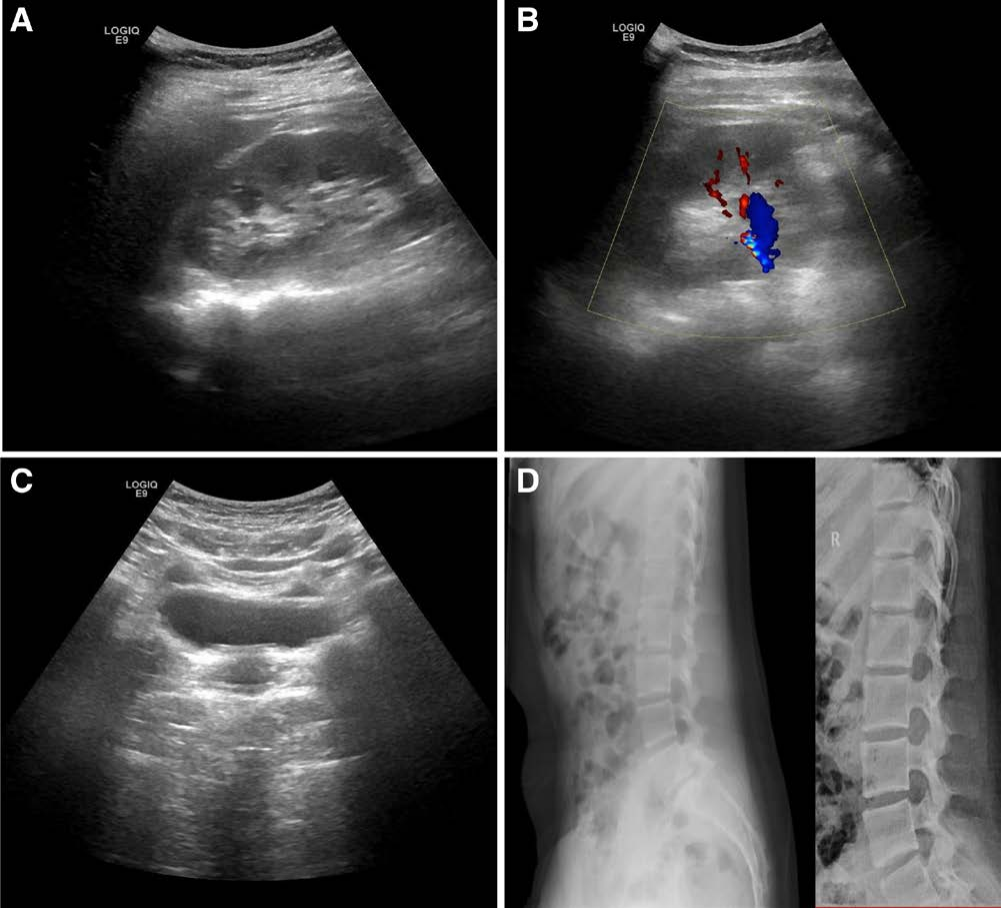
Urological system ultrasonic image and spine bone digital X-ray imaging. No abnormalities were detected. (A) Image of renal structure; (B) image of renal blood stream; (C) bladder image; (D) image of spine bone.
